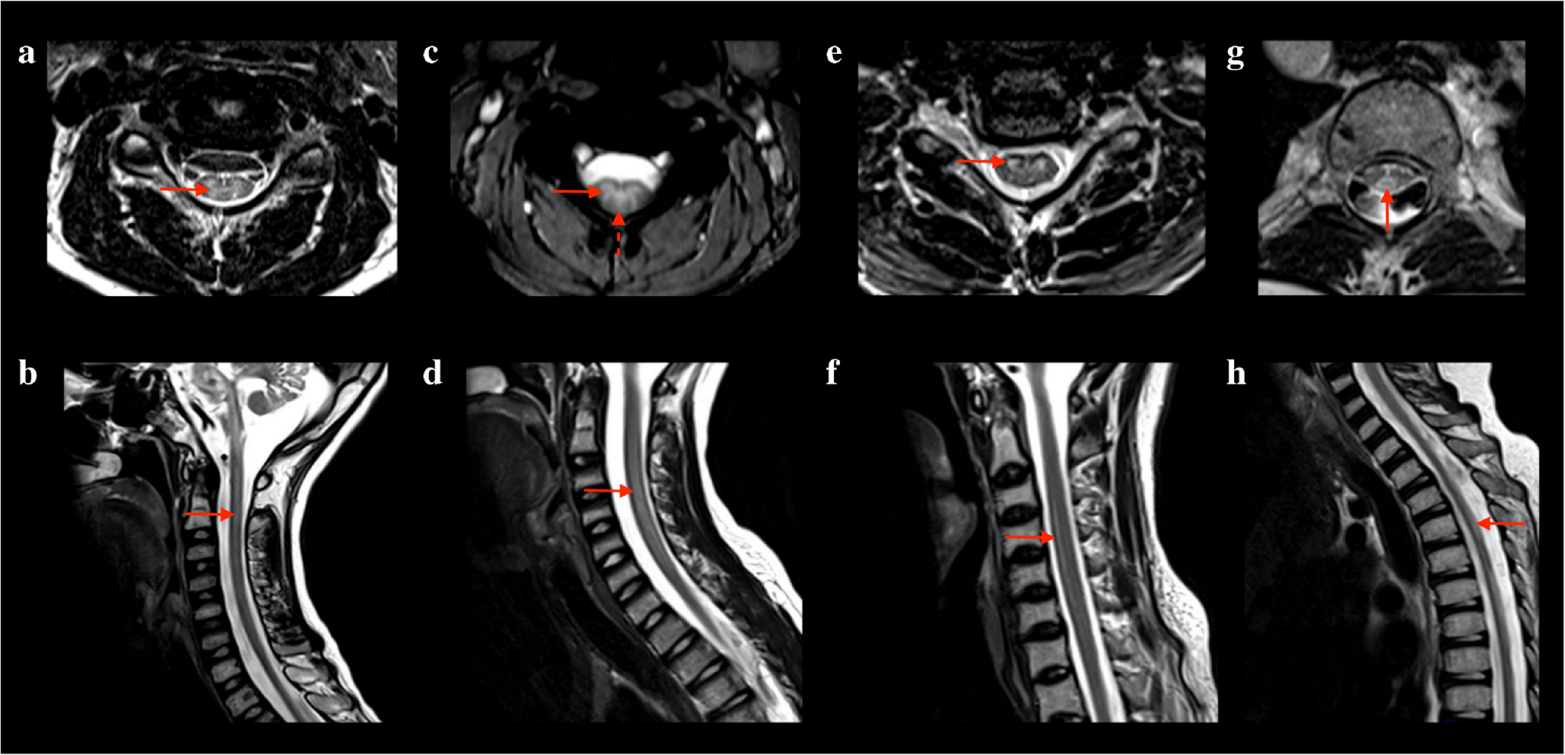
Axial and sagittal T2-weighted images displaying spinal cord disease in patients with KSS. Four predominant patterns were noticed: “H” pattern (solid arrows in image a and b); “H” plus pattern (image c and d, solid arrow in c pointing to gray matter and dotted arrow in c pointing to posterior columns); anterior pattern (solid arrows in e and f); posterior pattern (solid arrows in g and h)
